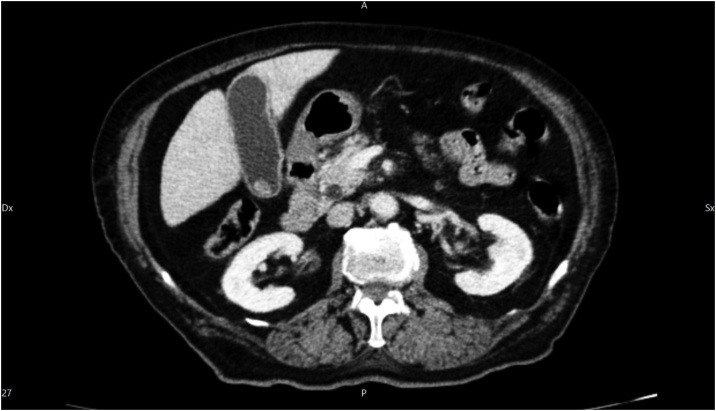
CT scan : gallbladder between left lobe and right lobe.
